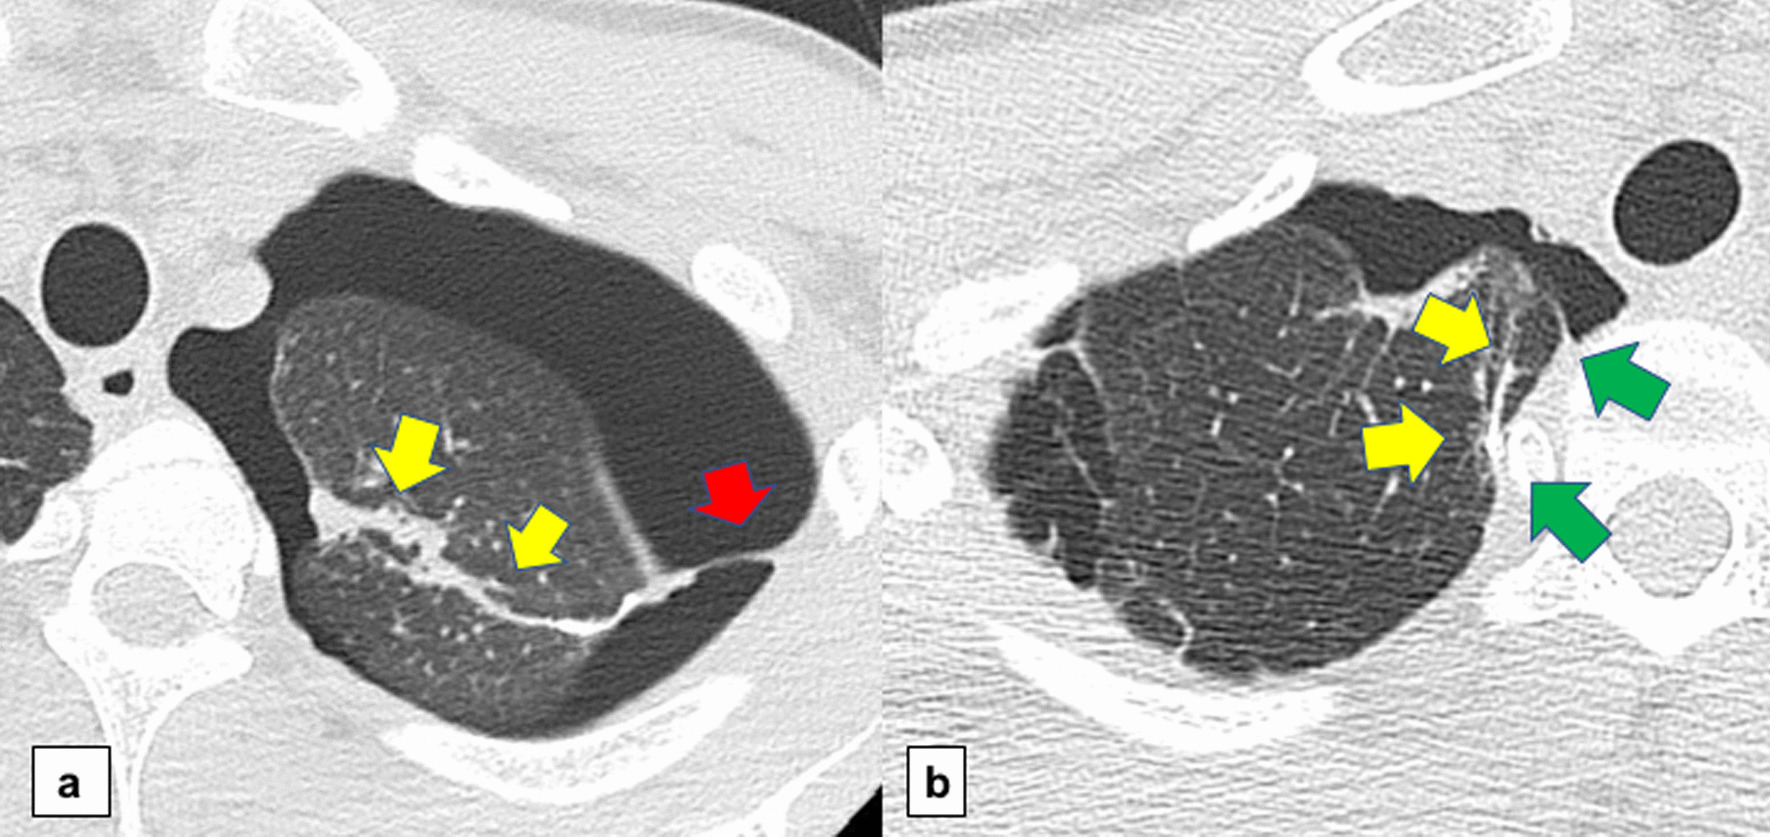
Computed tomography images at the time of recurrence. Yellow arrows indicate the staple line in each image. a. ORC coverage: only funicular adhesion (red arrow) was observed. b. PGA coverage: covered lung was tightly adhered to the chest wall, and green arrows indicate the thickened pleura. ORC, oxidized regenerated cellulose; PGA, polyglycolic acid
